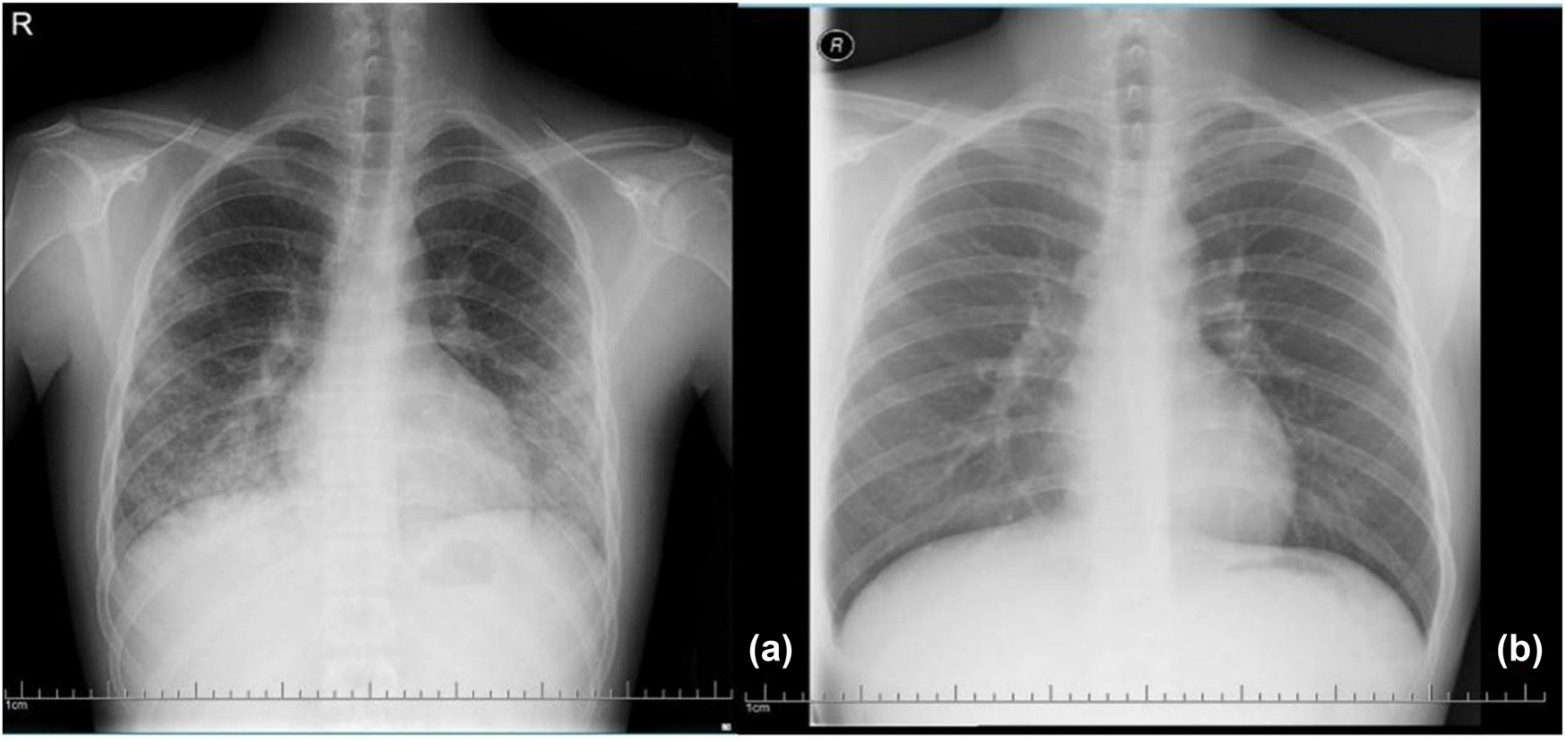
(a) Two days after admission, chest X-ray showed multiple plaques in both lungs. (b) After 5 months of treatment, no high-density shadows were observed on chest X-ray.
